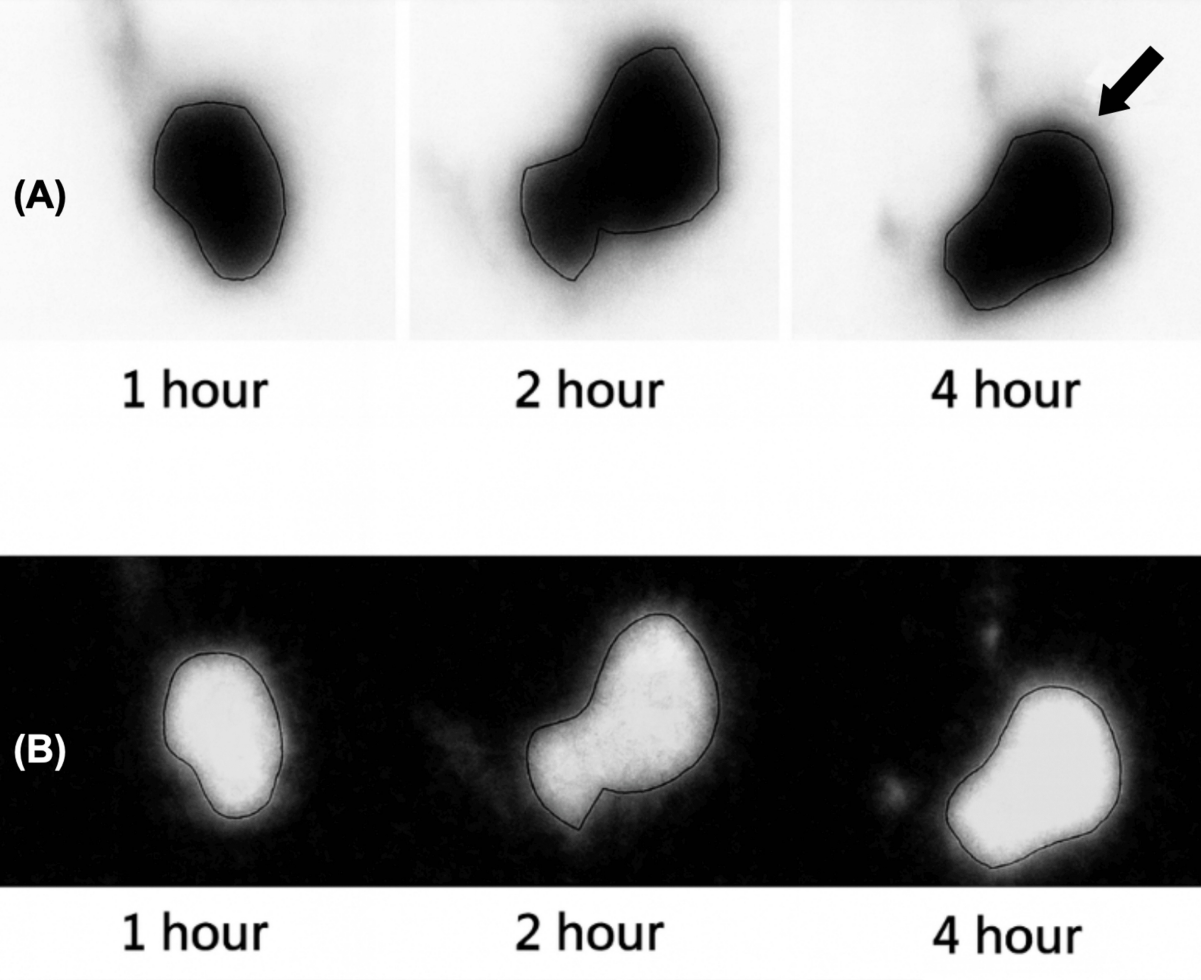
Serial gastric emptying scintigraphy. (A) Anterior gastric emptying images obtained at one, two, and four hours demonstrating progressive but incomplete clearance of radiotracer from the stomach. Persistent intragastric activity at four hours (arrow) exceeds expected normative retention thresholds, consistent with delayed gastric emptying. (B) Posterior gastric emptying images obtained at one, two, and four hours demonstrating delayed radiotracer clearance, supporting impaired gastric transit. These imaging findings align with mast cell-mediated dysmotility in the clinical context of mast cell activation syndrome.
